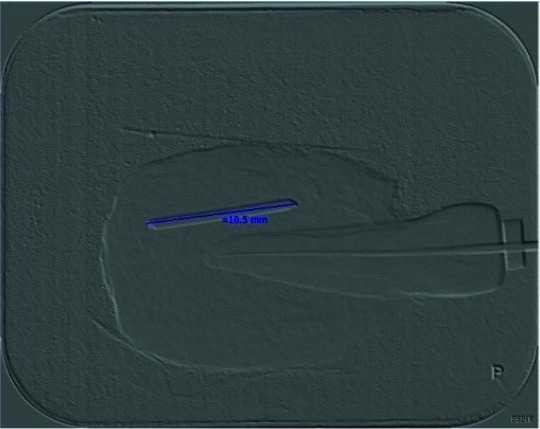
Digital radiograph with emboss filter
